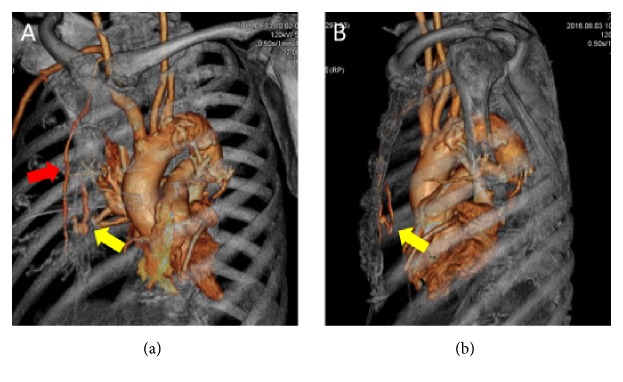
Three-dimensional contrast CT images. Left posteroanterior oblique view (a) and left-right view (b). The extravasation from a branch of IMA (yellow arrow) is confirmed. A red arrow shows the IMA main branch.
